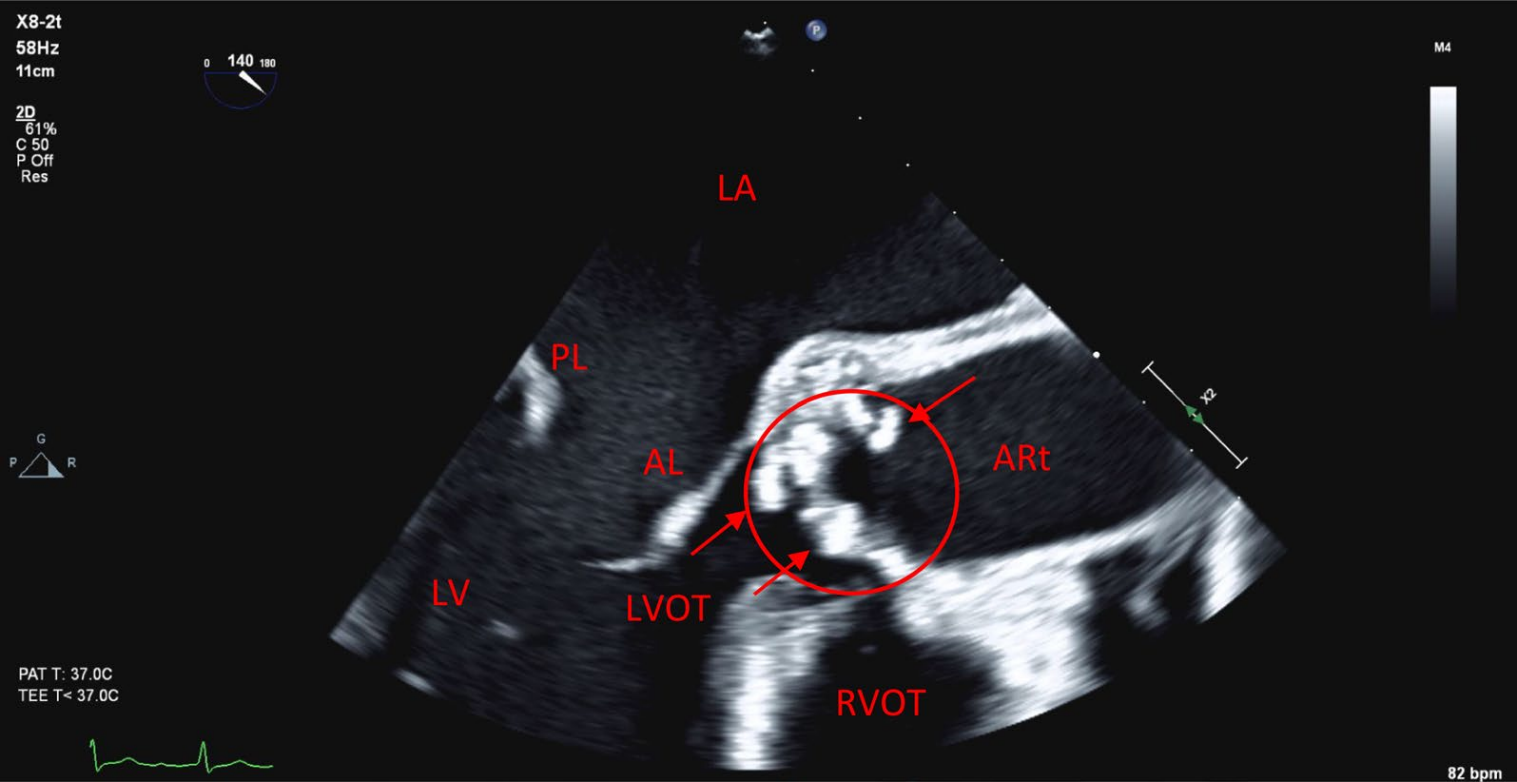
Transesophageal echocardiogram image showing a mid-esophageal long-axis view of the heart with an imaging plane angle of 140 degrees. Pertinent features of this still include the multiple hyperechoic structures on the aortic valve leaflets as indicated by the arrows. On real-time imaging, these were independently mobile of the valve leaflets and were deemed to be infective vegetations. For reference, other structures have been labeled, including left atrium (LA); posterior leaflet of the mitral valve (PL); anterior leaflet of mitral valve (AL); left ventricle (LV); left ventricular outflow tract (LVOT); aortic root (ARt); and right ventricular outflow tract (RVOT). Aortic valve is circled in red
